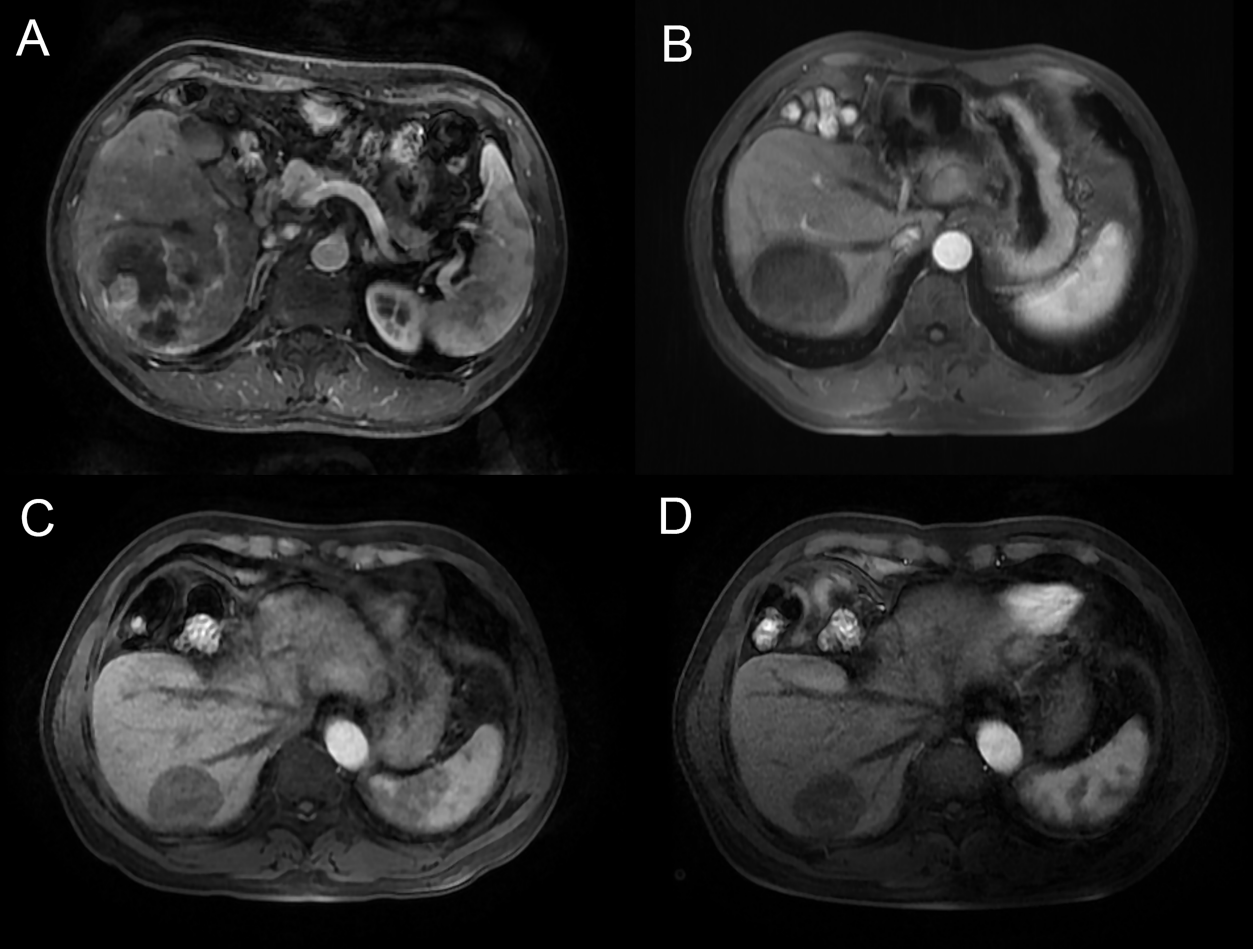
Male, 58y, BCLC B stage, Child-Pugh grade A6, massive and ruptured HCC. MR images (arterial phase) were acquired. (A) before TACE treatment, (B) 6 months after the combination treatment, (C) 12 months after the combination treatment, (D) 18 months after the combination treatment, demonstrating complete response, with a reduction in tumor size, the patient was classified as CR according to mRECIST. TACE, transarterial chemoembolization.
